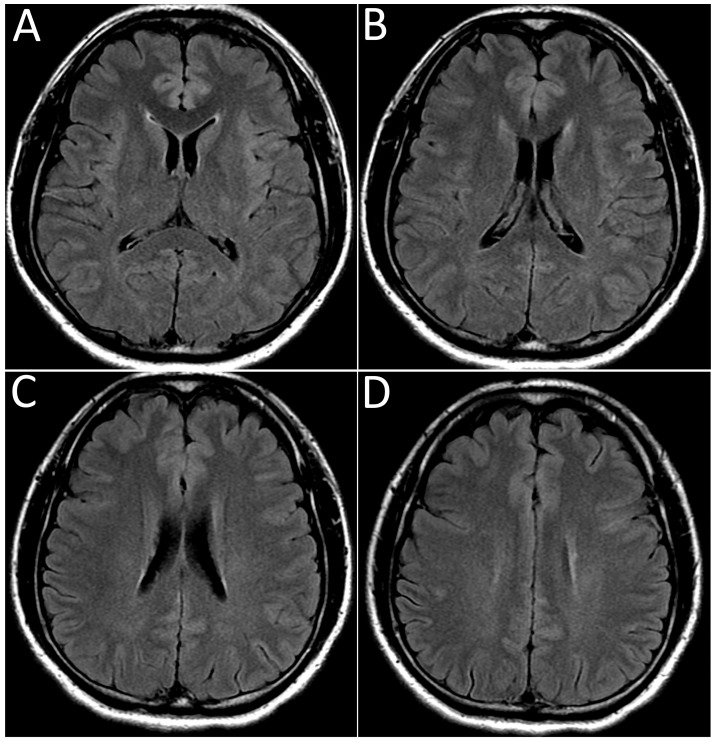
The serial axial MRI (A–D) of the brain on T2-weighted fluid-attenuated inversion recovery images showed no multiple sclerosis lesion in the periventricular area.
